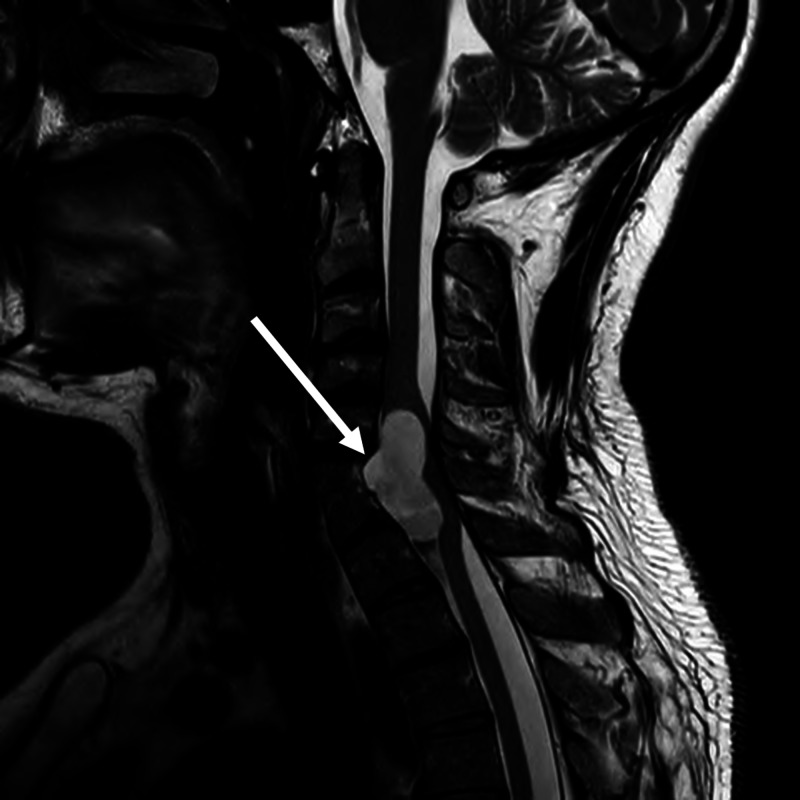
T2 MRI cervical spine demonstrating cystic lesion (white arrow) at C6/C7.
